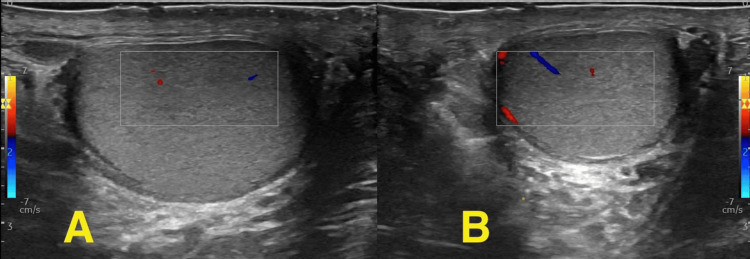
Doppler ultrasound Doppler ultrasound performed at the eighth postoperative month demonstrates normal blood flow in the right testis (A, right testis; B, left testis)
